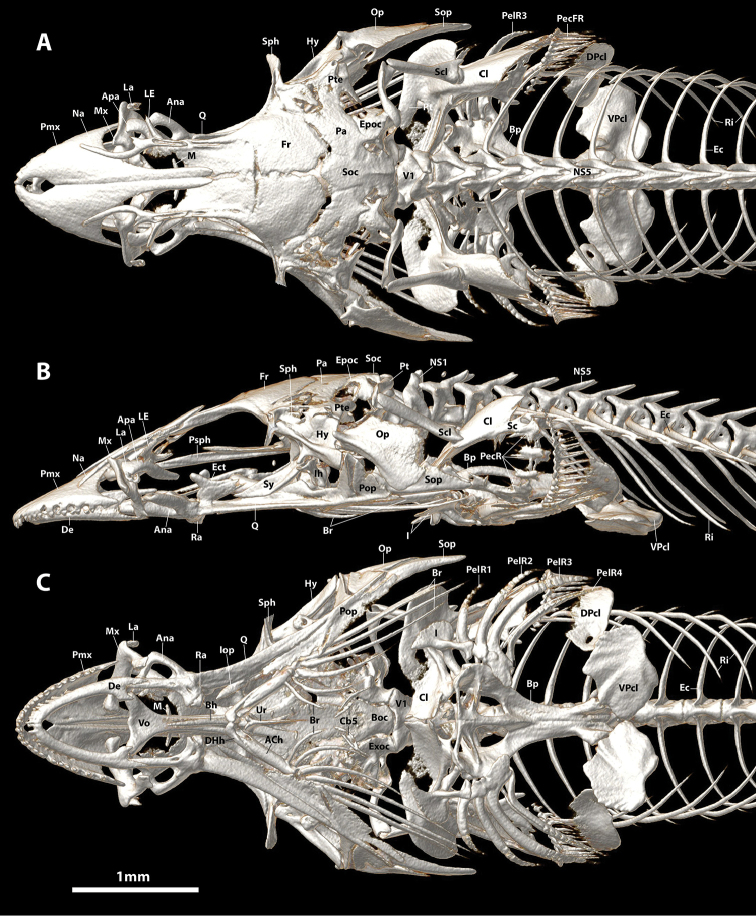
CT scanned anterior skeleton of *Aspasmichthysciconiae*, TCWC 16461.02, 26.0 mm SL. A Dorsal view B Lateral view (left side) C Ventral view. Abbreviations: ACh, anterior ceratohyal; Ana, anguloarticular; Apa, autopalatine; Boc, basioccipital; Bp, basipterygium; Br, branchiostegal rays; Cb5, ceratobranchial 5; Cl, cleithrum; DHh, dorsal hypohyal; DPcl, dorsal postcleithrum; Ec, epicentral; Ect, ectopterygoid; Epoc, epiotic; Exoc, exoccipital; Fr, frontal; Hy, hyomandibular; I, pelvic-fin spine; Iop, interopercle; La, lachrymal; LE, lateral ethmoid; M, mesethmoid; Na, nasal; NS1, 5, neural spine of vertebral centrum 1, 5; Op, opercle; Pa, parietal; PecR, pectoral radial; PecFR, pectoral-fin ray; PelFR1-4, pelvic-fin ray 1–4; Pop, preopercle; Pro, prootic; Psph, parasphenoid; Pt, posttemporal; Pte, pterotic; Q, quadrate; Ra, retroarticular; Ri, rib; Sc, scapula; Scl, supracleithrum; Soc, supraoccipital; Sop, subopercle; Sph, sphenotic; Ur, urohyal; V1, vertebral centrum 1; Vo, vomer; VPcl, ventral postcleithrum.
